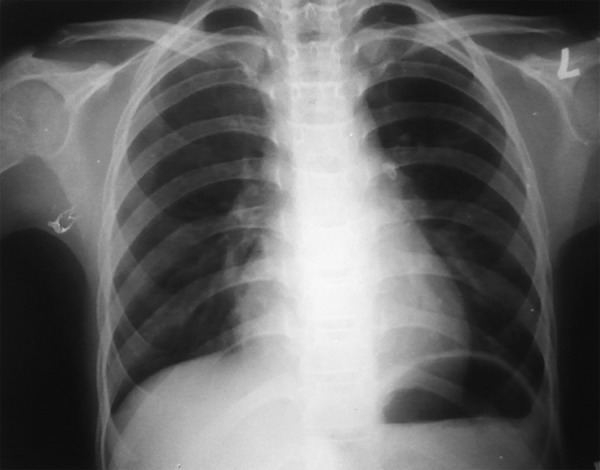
Radiograph showing cardiomegaly
